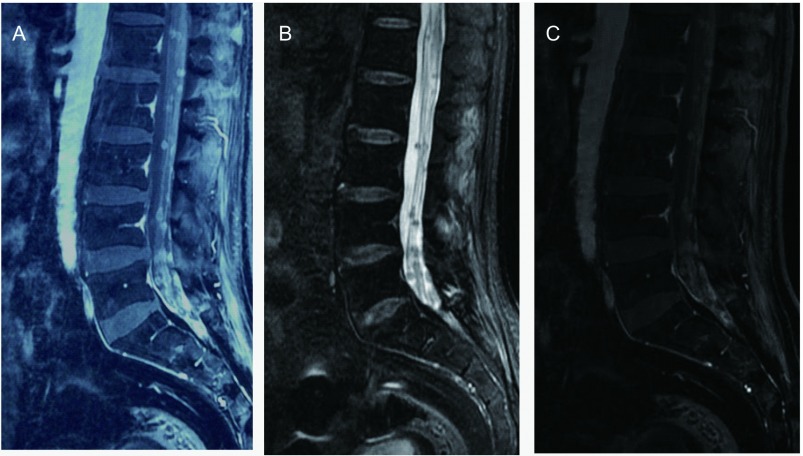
No.7患者腰椎MRI表现。A:T1WI椎管内脊髓外多发稍高信号软组织密度结节; B:T2WI椎管内脊髓外多发低信号的结节样充盈缺损; C:增强MRI显示椎管内脊髓外多发结节轻度强化。 Sagittal sections of MRI study of the lumbar spinal cord of patient No.7.A:T1-weighted imaging showed multiple hyperintense intradural extramedullary nodules; B:T2-weighted imaging showed multiple hypointense intradural extramedullary nodules; C:Contrast-enhanced MRI of lumbar spine showed multiple intradural extramedullary nodules with abnormal enhancement.
